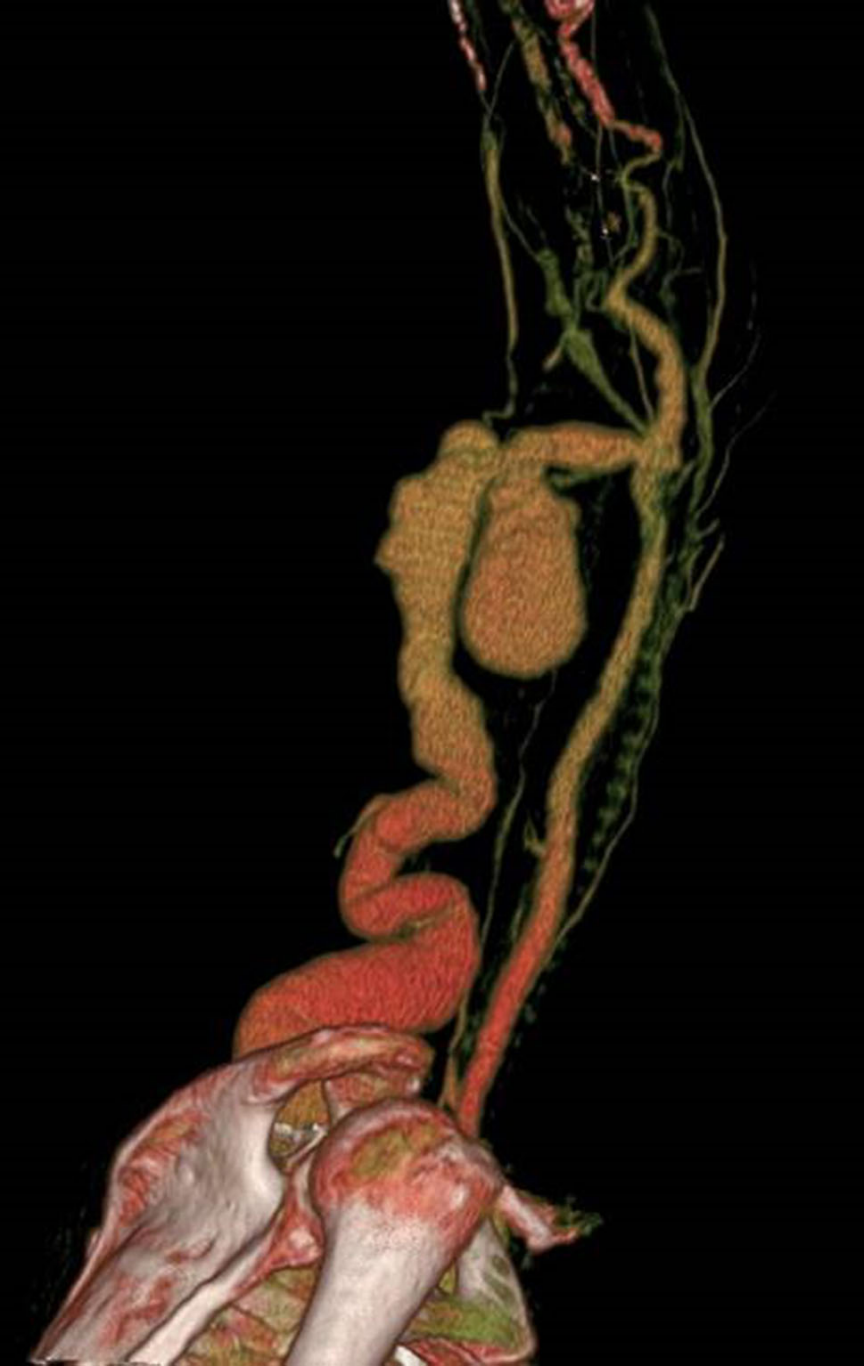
3D reconstruction CT scan of a left brachiocephalic AV Fistula. The patient presented with localized gross bruising and tenderness 24 h following dialysis. A pulsatile swelling was evident on clinical examination. Ultrasound examination was not possible due to patient discomfort. The image adequately demonstrates a large pseudoaneurysm arising from the proximal aspect of the AV fistula. This image provided vital pre-operative road mapping information to the surgeon before operating to repair this abnormality.
